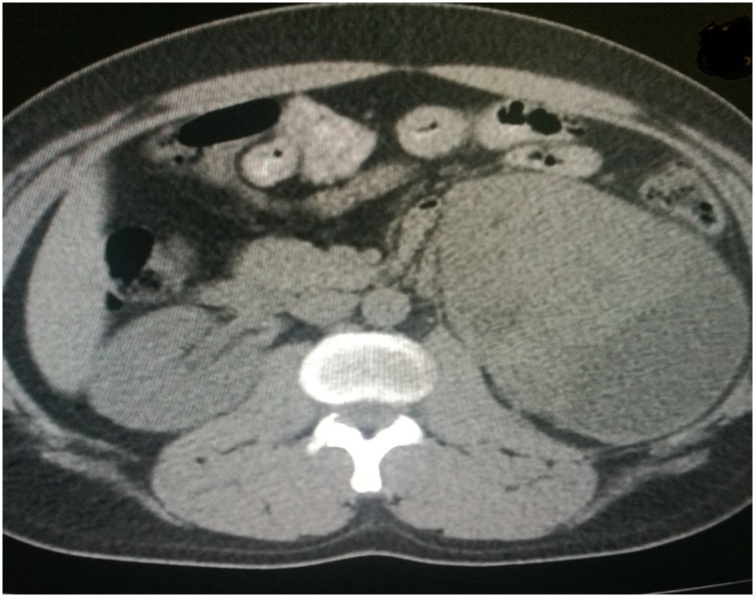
CT scan- axial view indicating left renal mass at the inferior pole.
